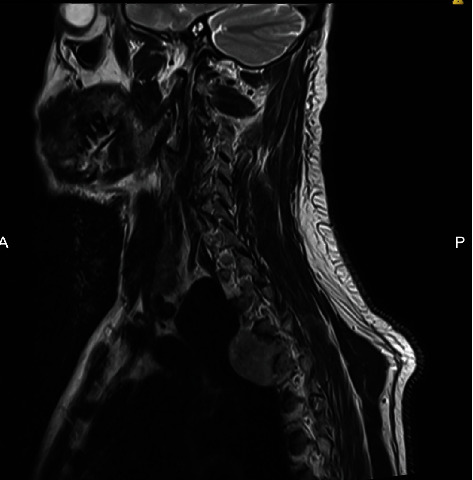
T2-weighted sagittal (view/section) MRI of the neck showing the pleural lesion.
